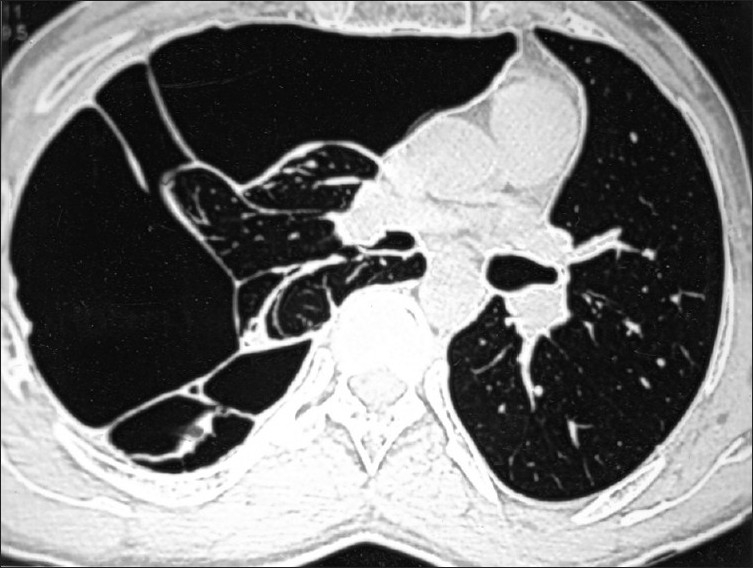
High-resolution computed tomography of thorax showing loculated multiseptate pneumothorax on the right side
